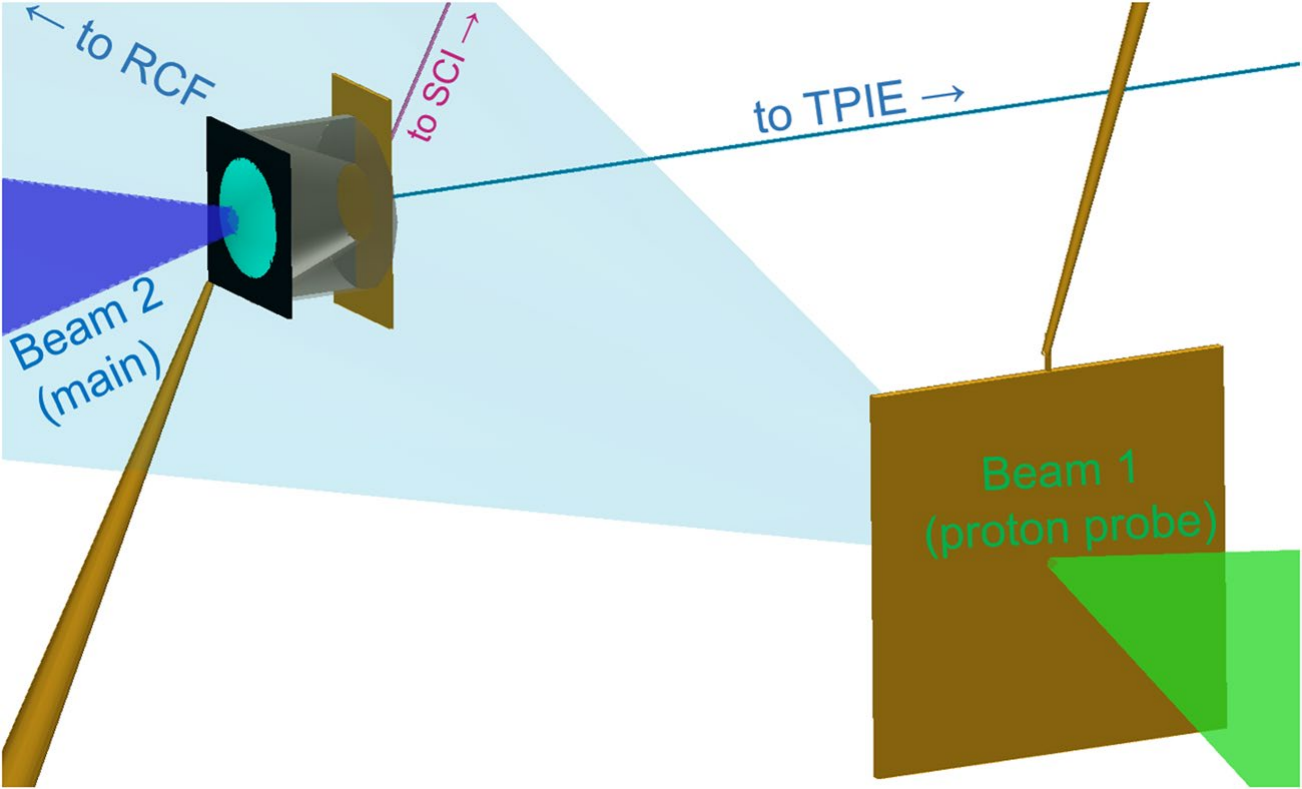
Experimental configuration drawn with VISRAD software. OMEGA EP short pulse main beam irradiated a curved diamond-like carbon target attached to an Al cone to produce an ultrahigh intensity proton beam, directed into various transport media. The cone is shown cutaway. A Cu diagnostic layer was glued to the back of the target. The other short pulse beam produced a transverse proton probing beam shown as a transparent cone pointing toward the RCF stack. Lines of sight toward TPIE and the SCI x-ray imager as shown as thin lines. Stalks supporting each target are shown as brown, tapered lines. For the freestanding case, a third stalk supported the Cu foil.
